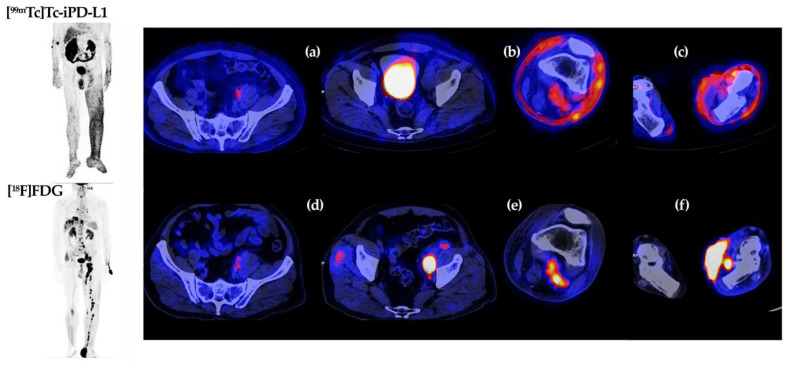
Molecular images of [^99m^Tc]Tc-iPD-L1 (top) and [^18^F]FDG (bottom) in a patient with plantar malignant melanoma. Axial plane at pelvic level: (a,d). Axial view of left lower leg: (b,e). Axial view of left foot (plantar lesion) and right foot: (c,f).
